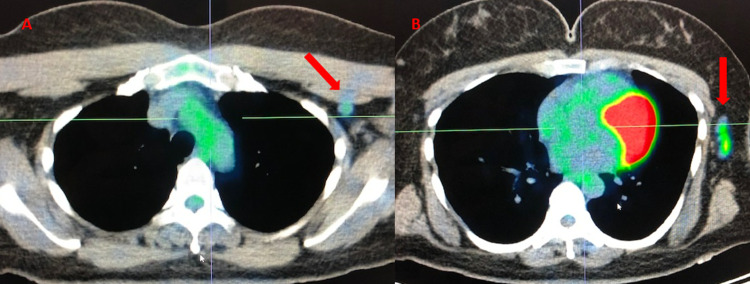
PET-CT six months after completion of debulking surgery and RT. PET-CT shows (A) hypermetabolic uptake in L subpectoral lymph node SUV 2.8 and (B) L axillary lymph nodes SUV 4.8 (red arrows). PET: positron emission tomography; RT: radiation therapy; L: left; SUV: standardized uptake value
